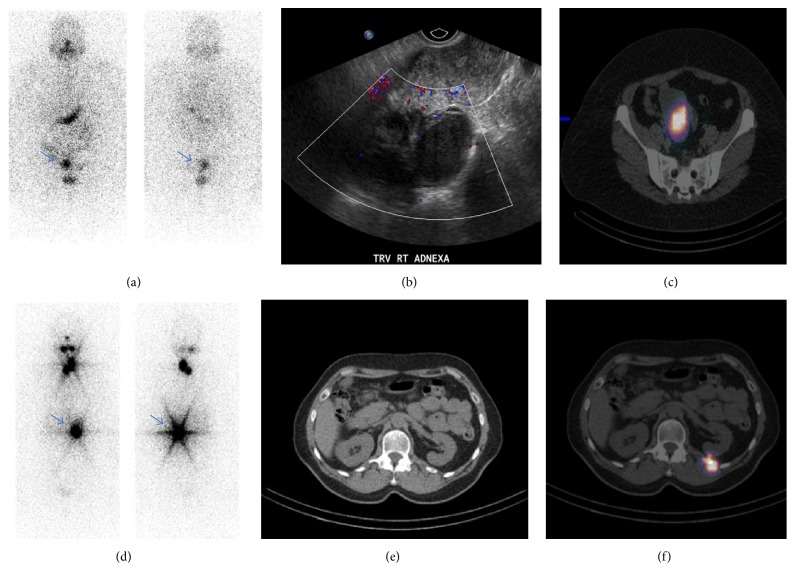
(a) Anterior and posterior planar views from a diagnostic I-131 WBS show focal uptake in the right pelvis (arrow). (b) Transverse sonographic image of the right adnexa shows a lobulated complex solid and cystic lesion with internal vascularity. (c) SPECT/CT localizes the area of radioiodine uptake to the right adnexal lesion, which on final pathology after surgical resection showed an endometrioma. (d) Anterior and posterior planar views from a postablation I-131 WBS show expected radiotracer uptake in the neck as well as an incidental focus of intense activity in the left lower thorax (arrow). (e) Axial CT image through the lower thorax shows a nondisplaced fracture of the left twelfth rib. (f) SPECT/CT localizes the area of radioiodine uptake to the left twelfth rib, which was biopsied and showed no evidence of malignancy.
